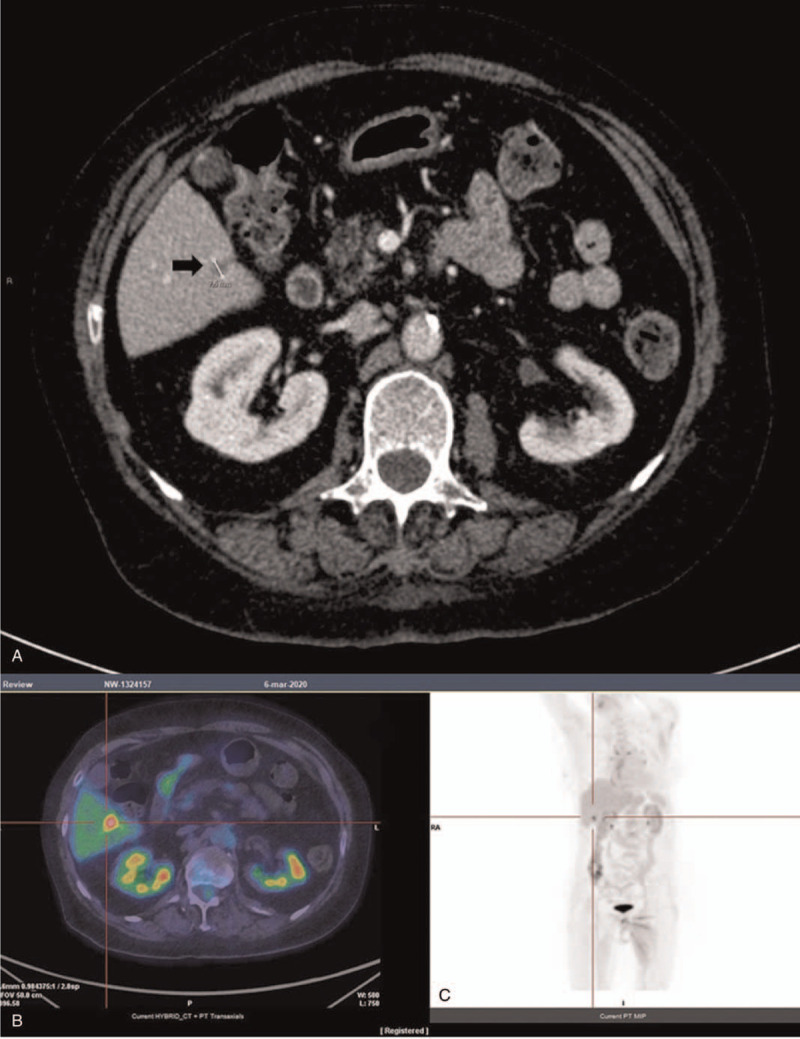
A, Axial contrast-enhanced T1-weighted MRI shows an enhancing hepatic mass (arrow); B and C, PET/CT images show an hepatic mass with FDG-avidity (arrows) (B, axial scan; C, coronal maximum-intensity-projection).
